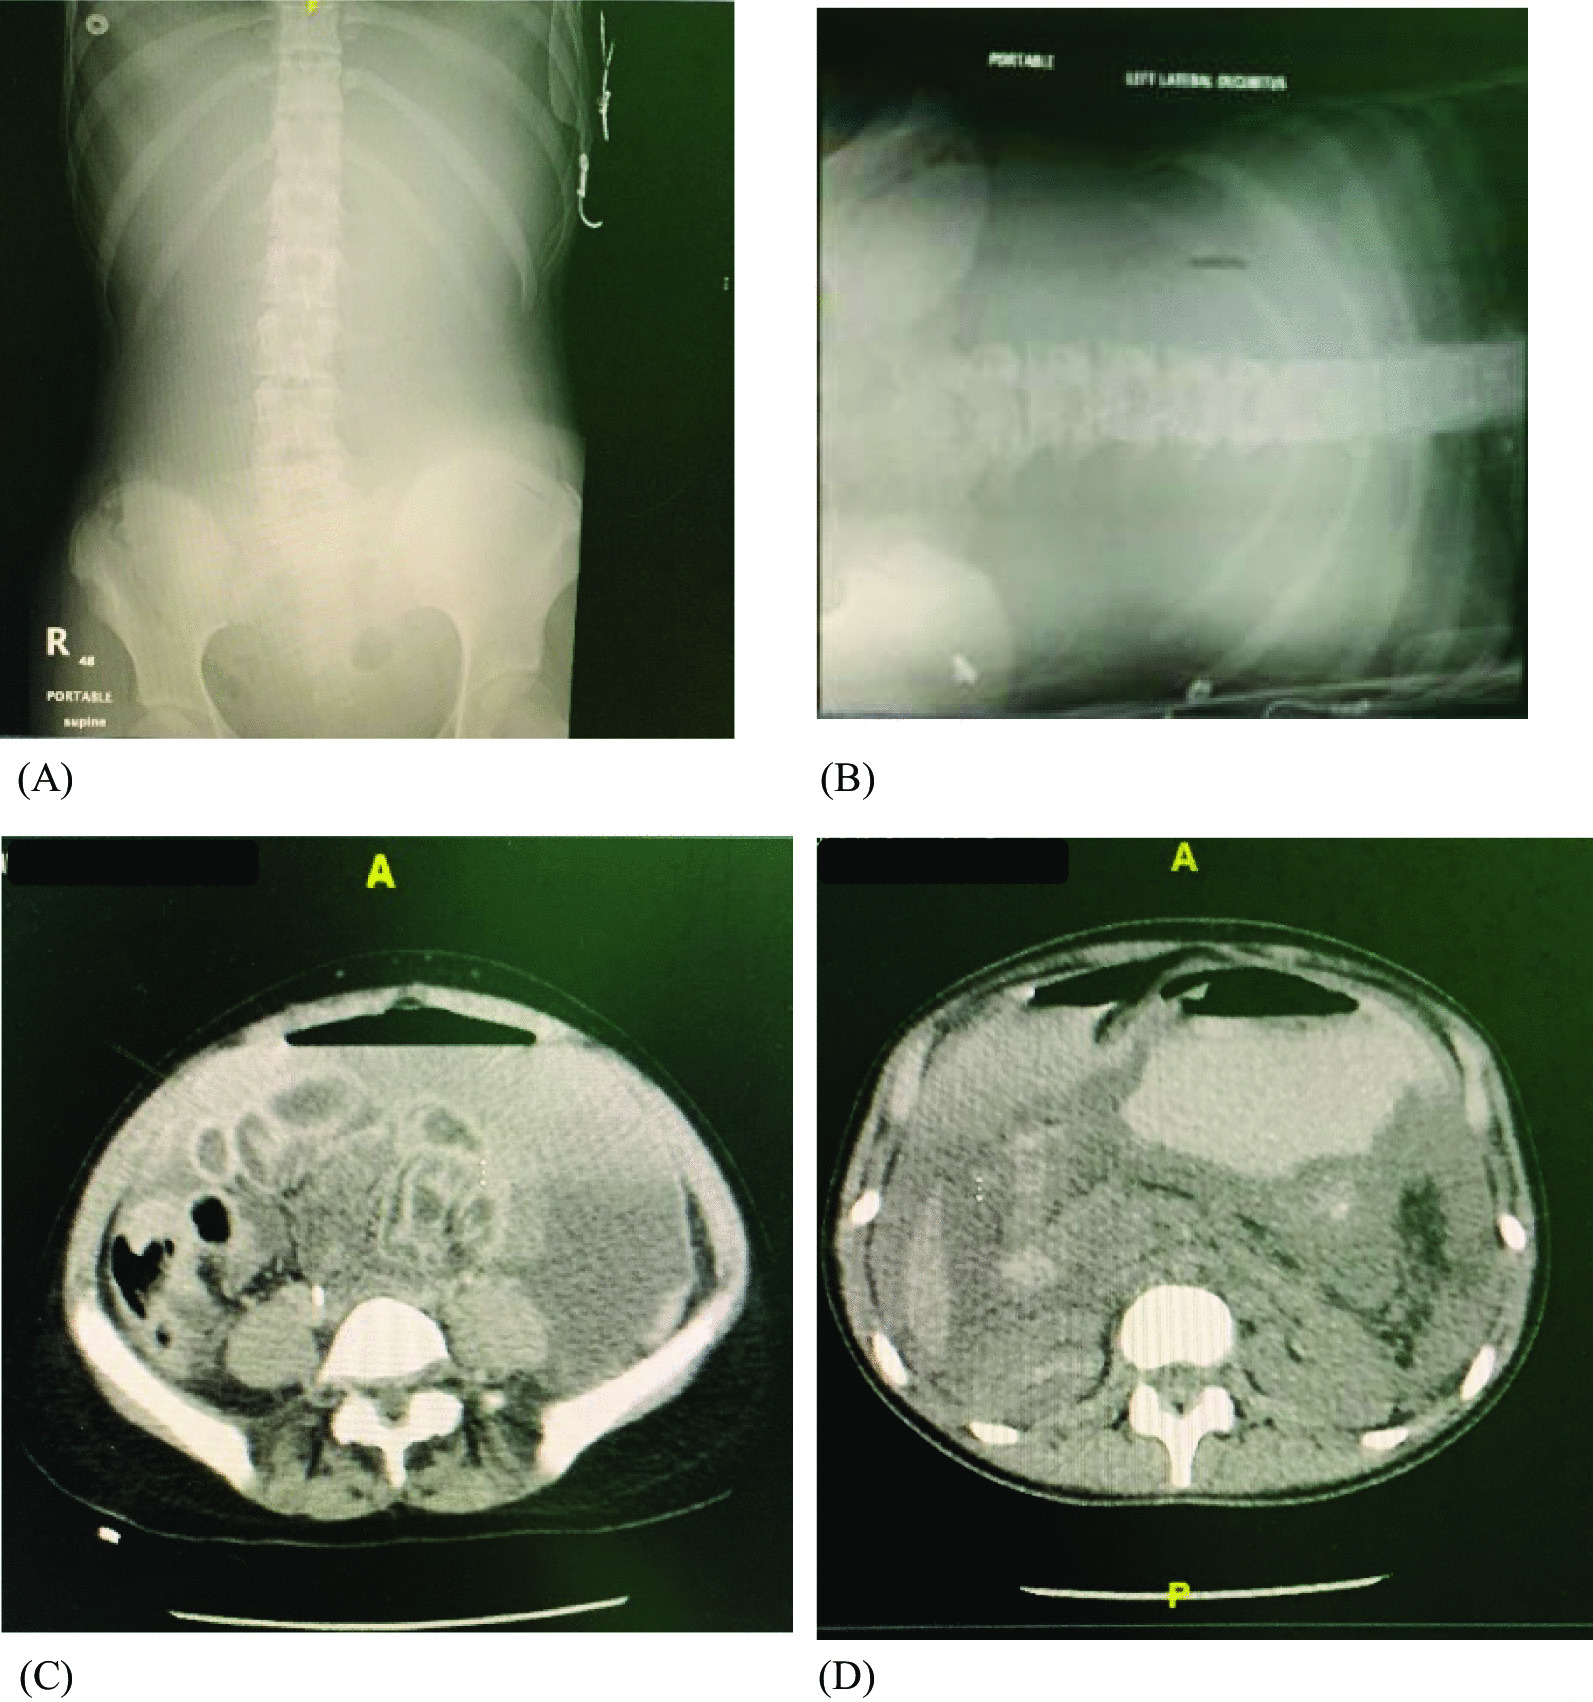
A Abdominal x-ray supine position; B lateral decubitus. C, D Computed tomography image of the abdomen and pelvis with contrast with findings of contrast extravasation
